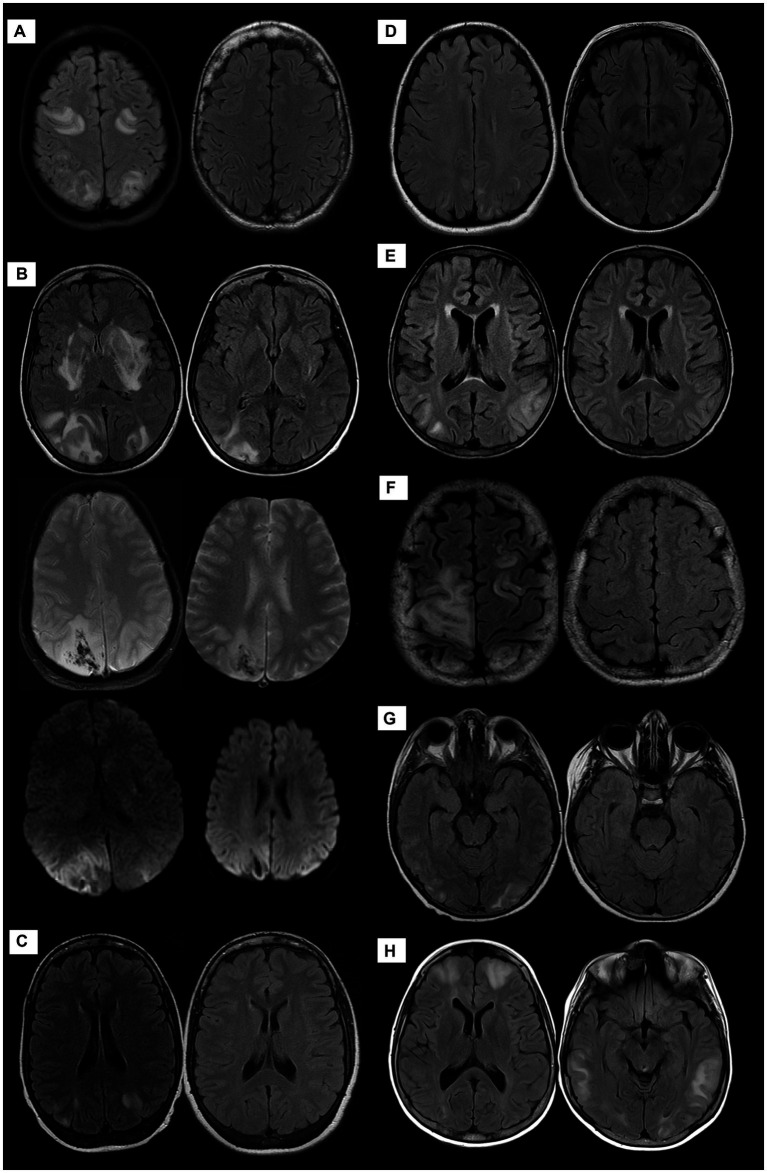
MRI of the brain of all patients demonstrating PRES manifestations at variable degrees; patient 1 (A): the initial exam of AXIAL FLAIR weighted images-(WI)-left side- shows predominantly sub-cortical signal alteration involving bilateral fronto-parietal and occipital lobes with complete resolution on the follow-up study right side. Patient 2 (B) Cortical and sub-cortical diffuse signal alteration at the bilateral parieto-occipital lobes with diffusion restriction and hemorrhage evident by blooming artifacts on SWI as well as unusual signal alteration at bilateral basal ganglia. Follow-up images at right side exhibit significant improvement with residual signal alteration of hemorrhagic PRES. Patient 3 (C), Patient 4 (D), and Patient 5 (E): AXIAL FLAIR WI demonstrate predominantly sub-cortical signal alteration involving bilateral parieto-occipital lobes with complete resolution on follow-up images right side except for patient 4, no follow-up images. Patient 6 (F), Patient 7 (G), and Patient 8 (H) AXIAL FLAIR WIs demonstrate predominantly sub-cortical white matter signal alteration at different distributions, mainly parieto-occipital to a lesser extent involving fronto-temporal lobes with complete resolution on follow-up images right side except for patient 8, no follow-up images.
